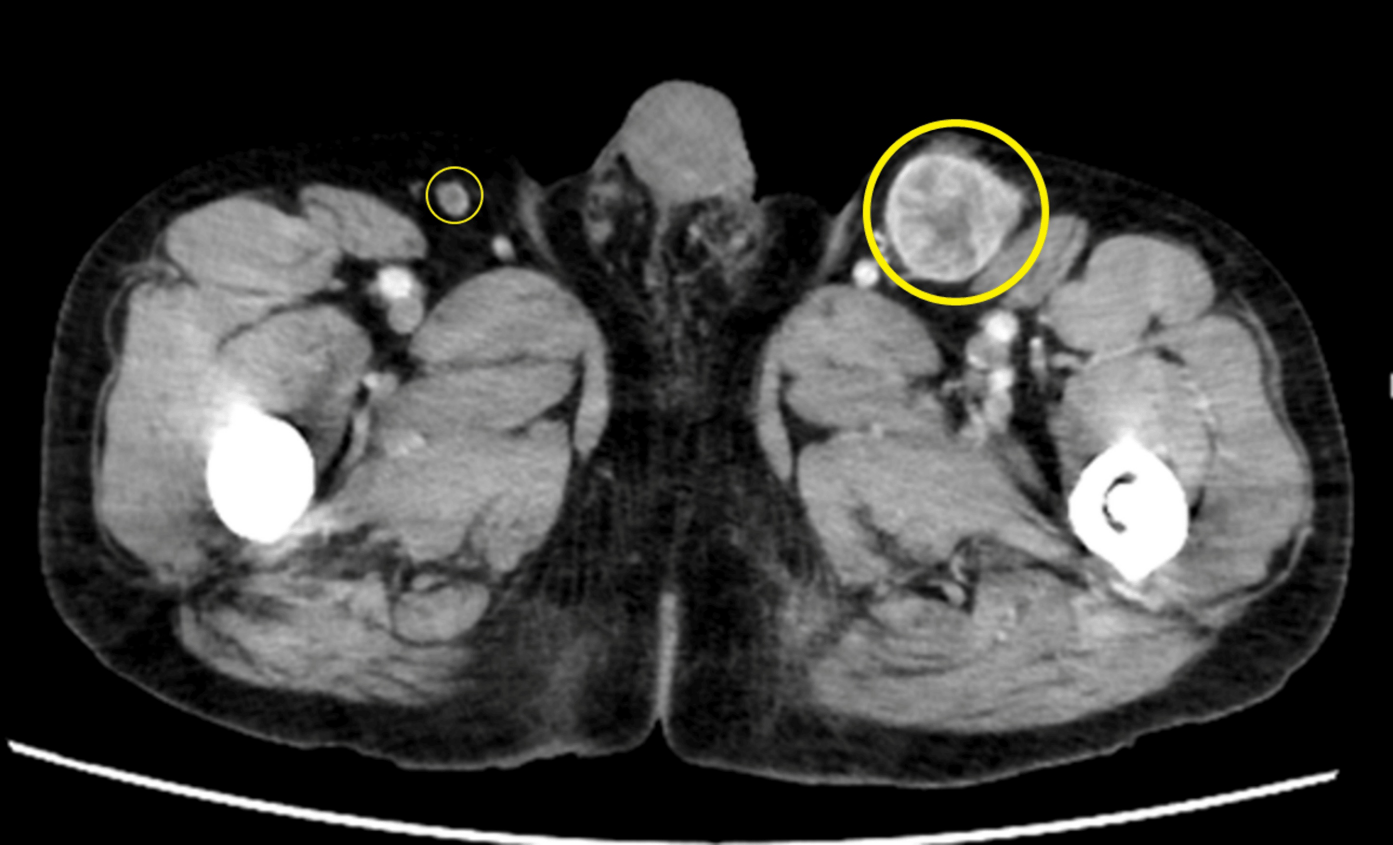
Computed tomography. A partially contrasted mass in the left inguinal area and a small mass (7 mm) were observed in the right inguinal area.
